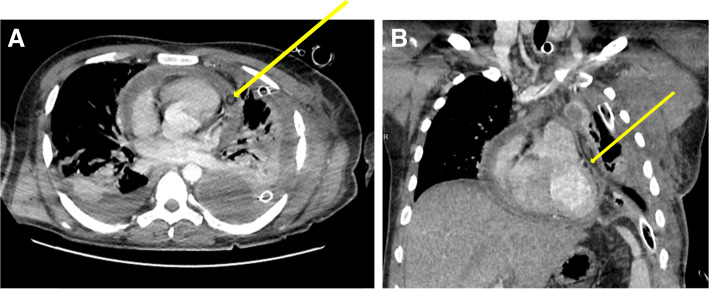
Chest CT performed before intrapericardial r-tPA treatment. Purulent pericarditis and pleuritis in the course of common variable immunodeficiency syndrome. Pericardial effusion, the layer of 17 mm. Thickening of the pericardium up to 4 mm. Drain in the pericardial sac - arrow. Bilateral pleural effusion, drain in the left pleura. Large atelectasis in the lower parts of the lungs due to pleural effusion. a – Mediastinal window, axial view. b – Mediastinal window, frontal view
